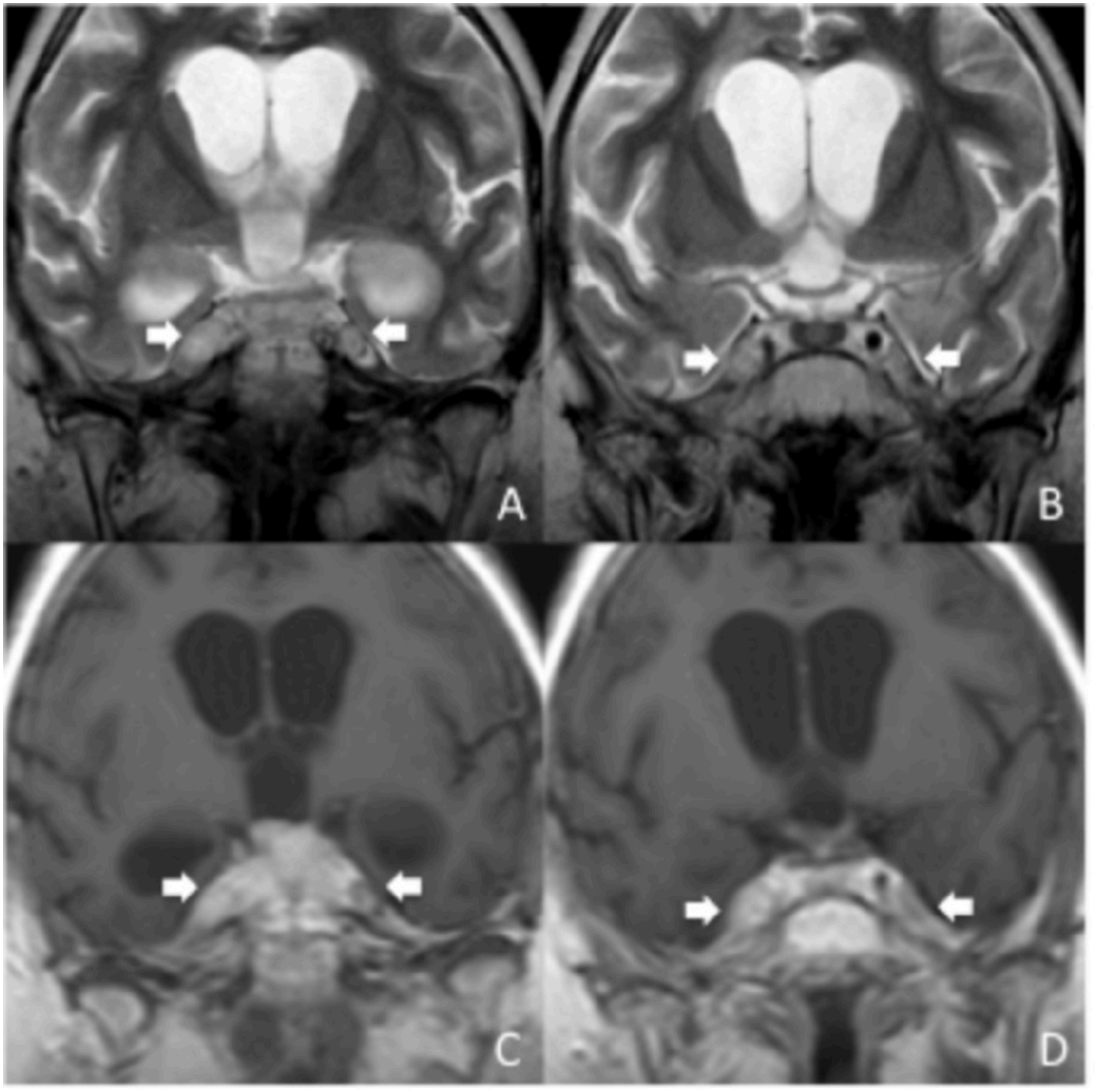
MRI findings of V cranial nerve involvement at follow-up in a 4-years-old girl previously diagnosed with DIPG. Coronal TSE T2 (A,B) and post-contrast coronal T1 (C,D) images show extensive thickening of both V cranial nerves and abnormal bulging of the Meckel caves, consistent with direct infiltration by the tumor (A–D, white arrows). There is associated contrast-enhancement (C,D, white arrows).
